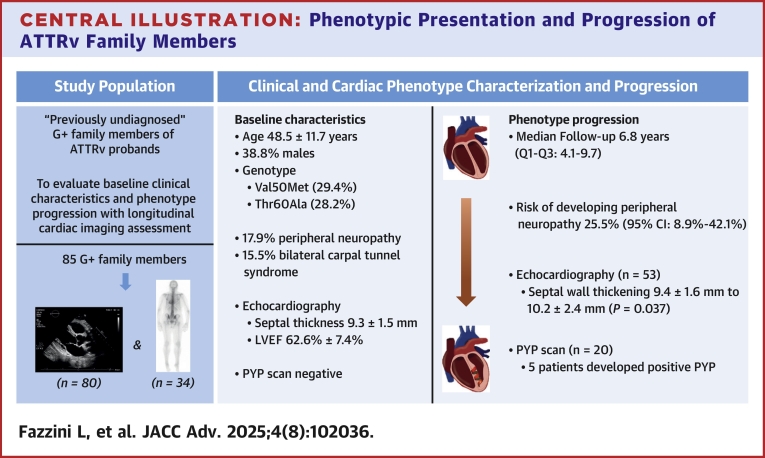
Phenotypic Presentation and Progression of ATTRv Family Members We explored the study population’s baseline features and longitudinal findings in asymptomatic ATTRv family members. At baseline, individuals had normal echocardiographic and pyrophosphate scan profiles. Over the follow-up, we described clinical and echocardiographic progression. In patients with follow-up bone scintigraphy, 5 developed a positive scan. This study highlights the features of progression over time and supports the importance of structured surveillance in *TTR* variant carriers. ATTRv = hereditary transthyretin amyloidosis; *TTR* = transthyretin.
